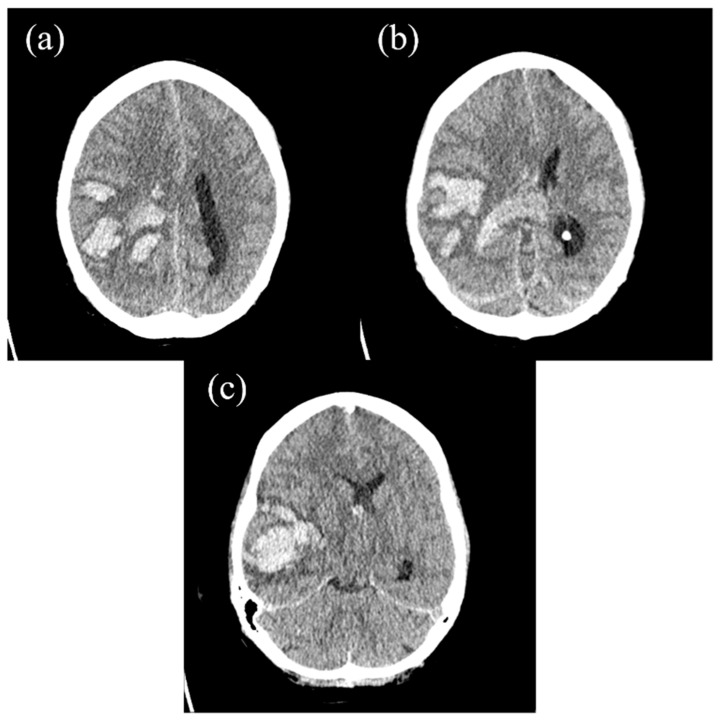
(a–c) The axial cranial CT scan showing a massive, multilocular ICH in the right hemisphere, causing a significant midline shift due to the local mass effect and intraventricular hemorrhage.
